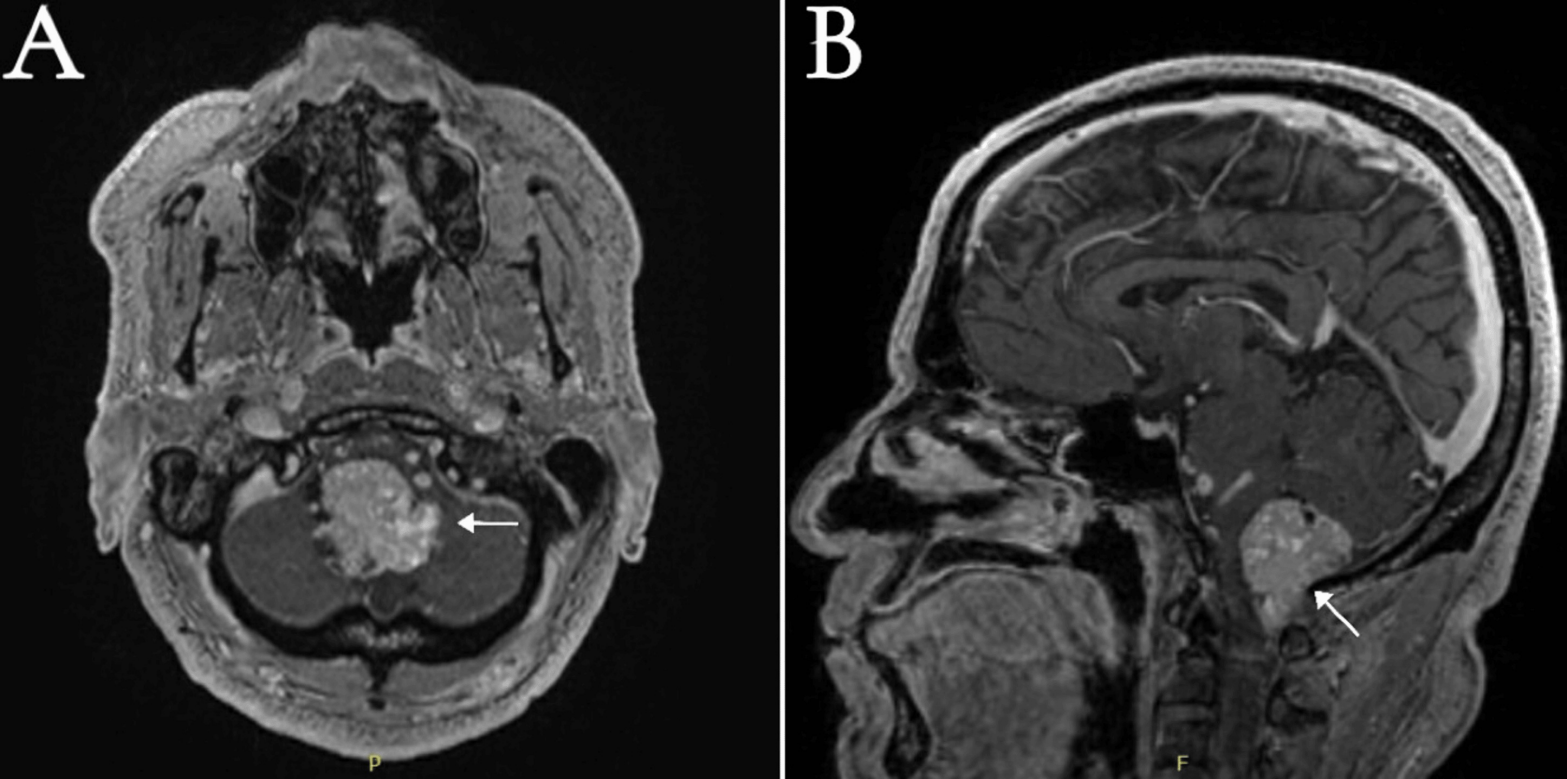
Preoperative post-contrast T1-weighted MRI of the brain: (A) axial and (B) sagittal. The image reveals contrast vividly enhancing an intradural extra-axial solid mass (white arrows) measuring 3.3 × 3.0 × 4.5 cm in the transverse anteroposterior and craniocaudal diameters at the cervicomedullary junction. Multiple large vessels can be noticed within and surrounding the mass in keeping with multiple arterial feeders from the posterior circulation, exerting a significant mass effect on the brainstem and upper cervical cord.
